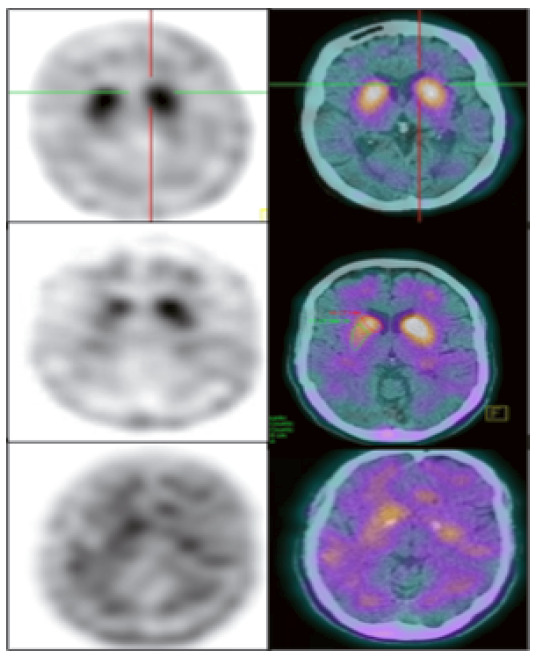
Dopamine transporter uptake in basal ganglia shown by SPECT or PET. Dopamine transporter uptake in basal ganglia demonstrated by TRODAT-1 SPECT imaging. The superior row shows a normal uptake in the caudate and putamen. The medium row shows an asymmetric uptake in a PD patient. The lower row shows a bilateral minimal uptake in a DLB patient. (Reproduced with permission from Dr. Artur Coutinho, Nuclear Medicine Center - Inrad - HC-FMUSP).
